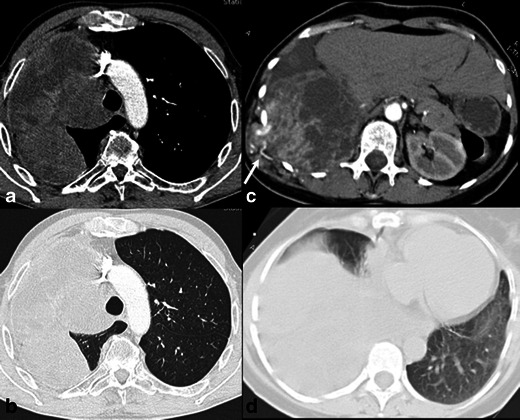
CT images of malignant solitary fibrous tumour. A 76-year-old man (a and b) with presenting as a large lesion, with irregular shape, lobulated margins and important necrosis. A 60-year-old woman (c and d) with malignant solitary fibrous tumour, with a mass presenting necrosis, intratumoural vessels, infiltration of the chest wall, and associated prominent collateral feeding vessels also seen outside the tumour (*arrow* in c)
